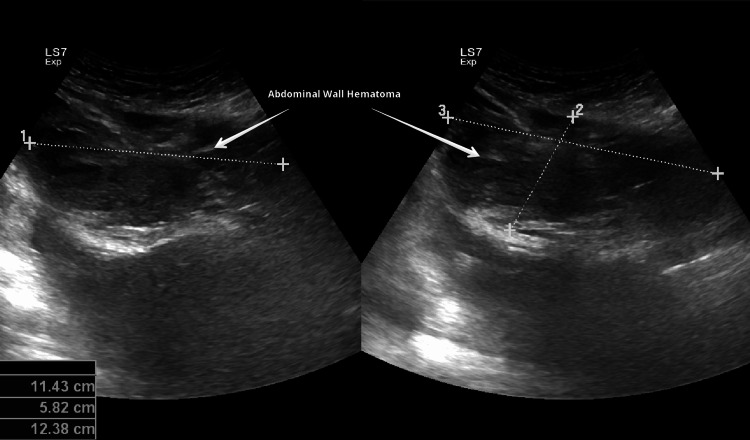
Ultrasound image shows midline infraumbilical hematoma of rectus sheath of size 11.4 cm x 5.8 cm x 12.3 cm.
